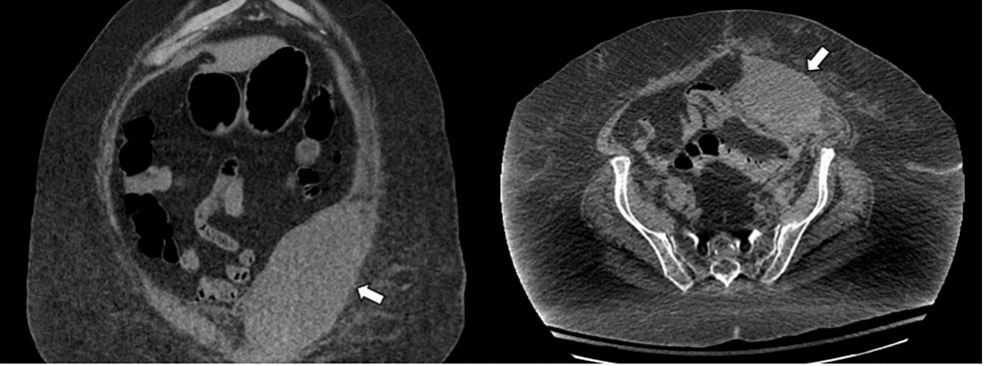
Large Rectus Sheath Hematoma Coronal and axial CT of the abdomen shows a large left rectus sheath hematoma measuring 17.2 x 9.3 cm which deviates the bladder toward the right (arrow).
